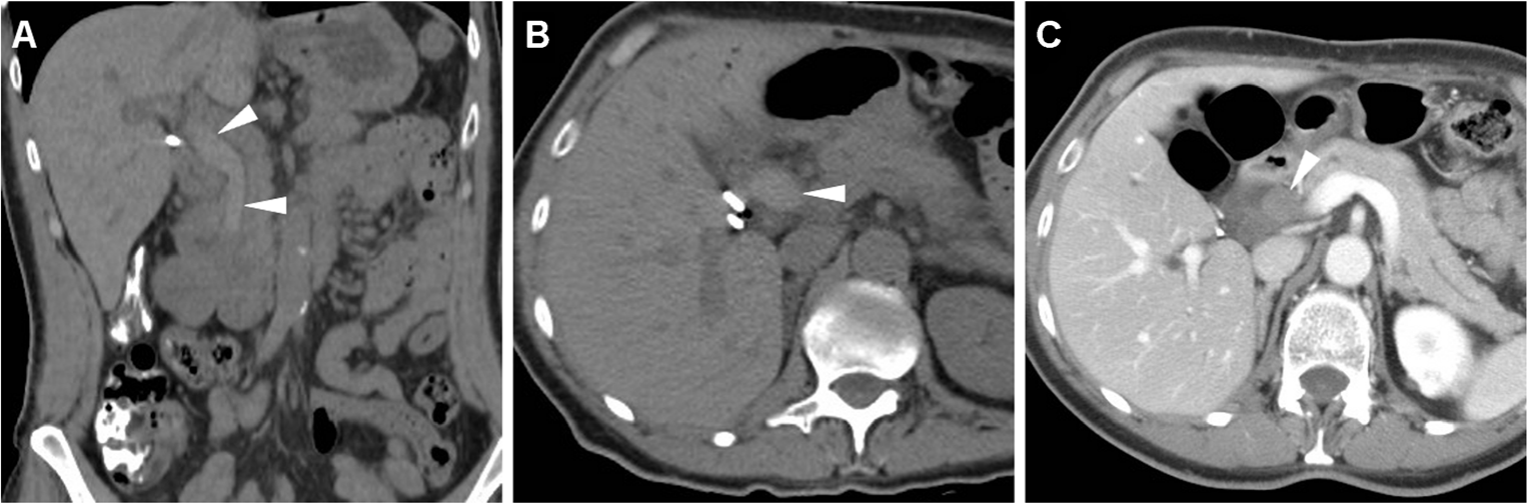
Haemobilia following ERCP in a 57-year-old female with benign papillary stenosis and previous cholecystectomy, manifesting with abdominal pain and mild haemoglobin drop. Unenhanced (a, b) and post-contrast (c) CT images showed moderate dilatation and hyperattenuating (45 HU) content of the CBD (*arrowheads*) corresponding to endoluminal haemorrhage but initially misinterpreted as retained CM injected during the procedure, without signs of active bleeding. This complication was self-limiting and resolved on conservative treatment
